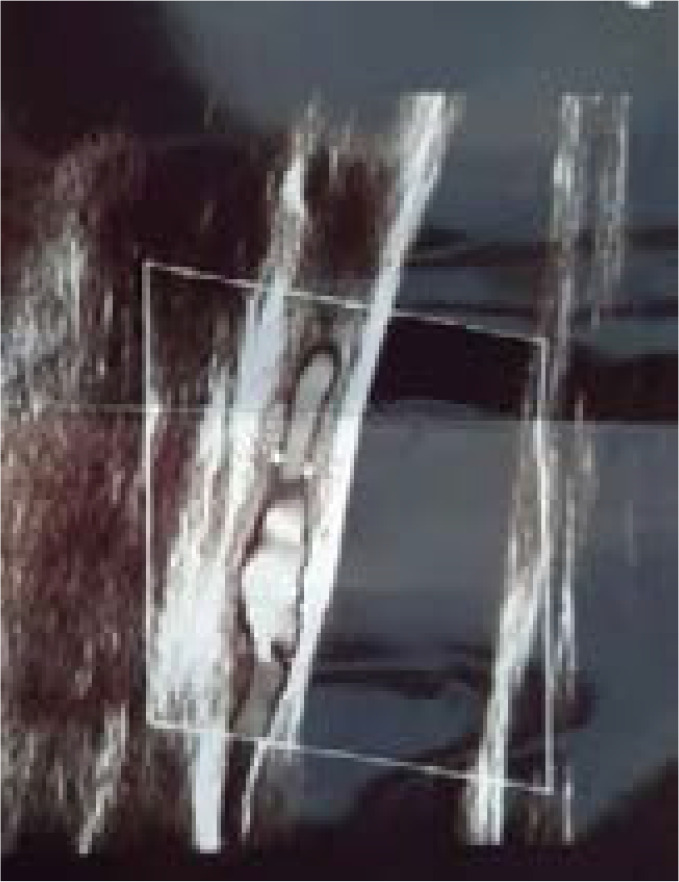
Doppler ultrasound of the left vertebral artery showing reverse flow (subclavian steal syndrome)
